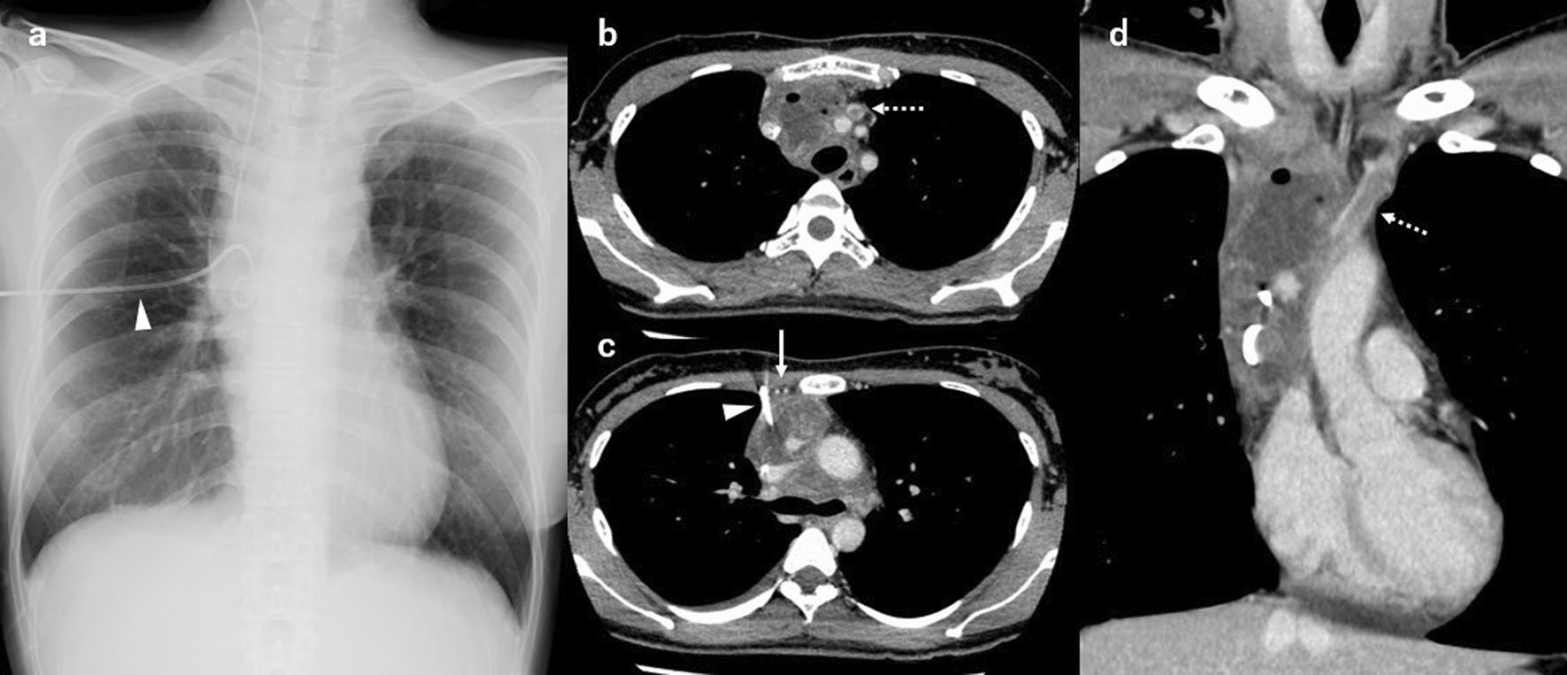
Chest X-ray image (a) and contrast-enhanced CT findings (b–d) after extubation. The image b is at the trachea level. The image c is at the level of carina of the trachea. The image d is the coronal section. The pigtail catheter (white arrowhead) was inserted laterally into the right internal thoracic artery and vein (white arrow). The left brachiocephalic vein (dotted white arrow) was surrounded by the tumor and thrombosed
